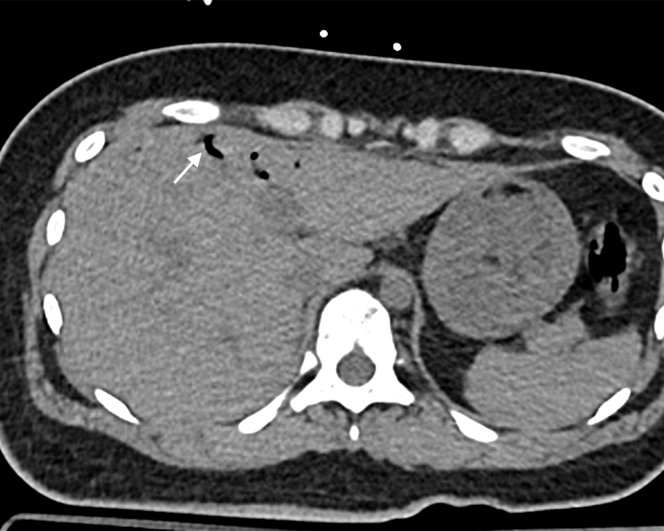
Axial abdominal computed tomography: hepatic venous pneumatosis (arrow, left hepatic vein). Focal gas foci in the right hepatic vein confirmed hepatic venous pneumatosis, a core component of porto-mesenteric venous gas.3.Coronal abdominal CT: linear gas tracks in superior mesenteric vein branches extended PMVG beyond the hepatic veins, ruling out isolated venous gas and suggesting more extensive gastrointestinal involvement (Fig 3).
